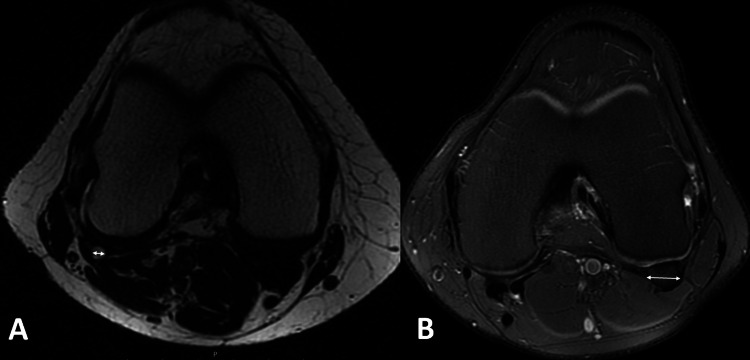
T2 (A) and axial proton density fat-saturated (B) MRI views demonstrating the measurement of fabella’s width using the double arrow.
